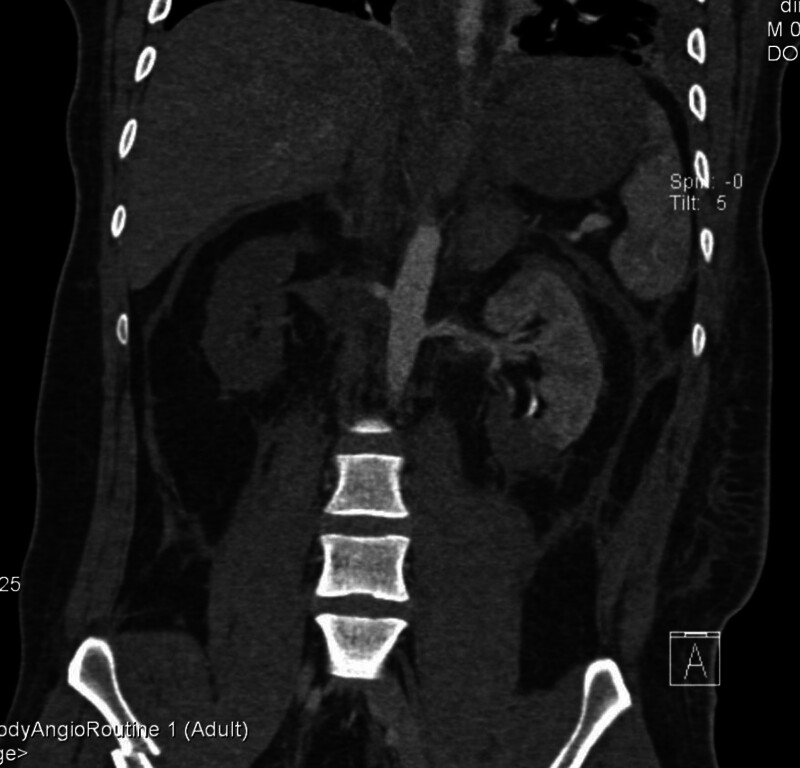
CTA demonstrates poor visualization of the right kidney with focal ischemia in the lower pole of the left kidney.
